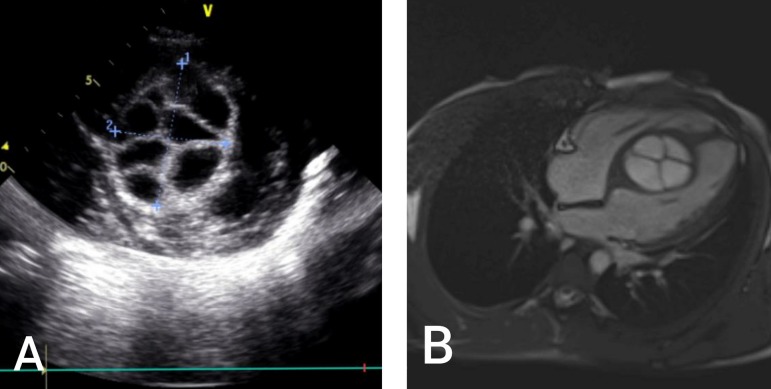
A)Transthoracic echocardiography showing the hydatid cyst in the interventricular septum; B) cardiac magnetic resonance ımaging showing the huge interventricular cyst partially obstructing both ventricles.
